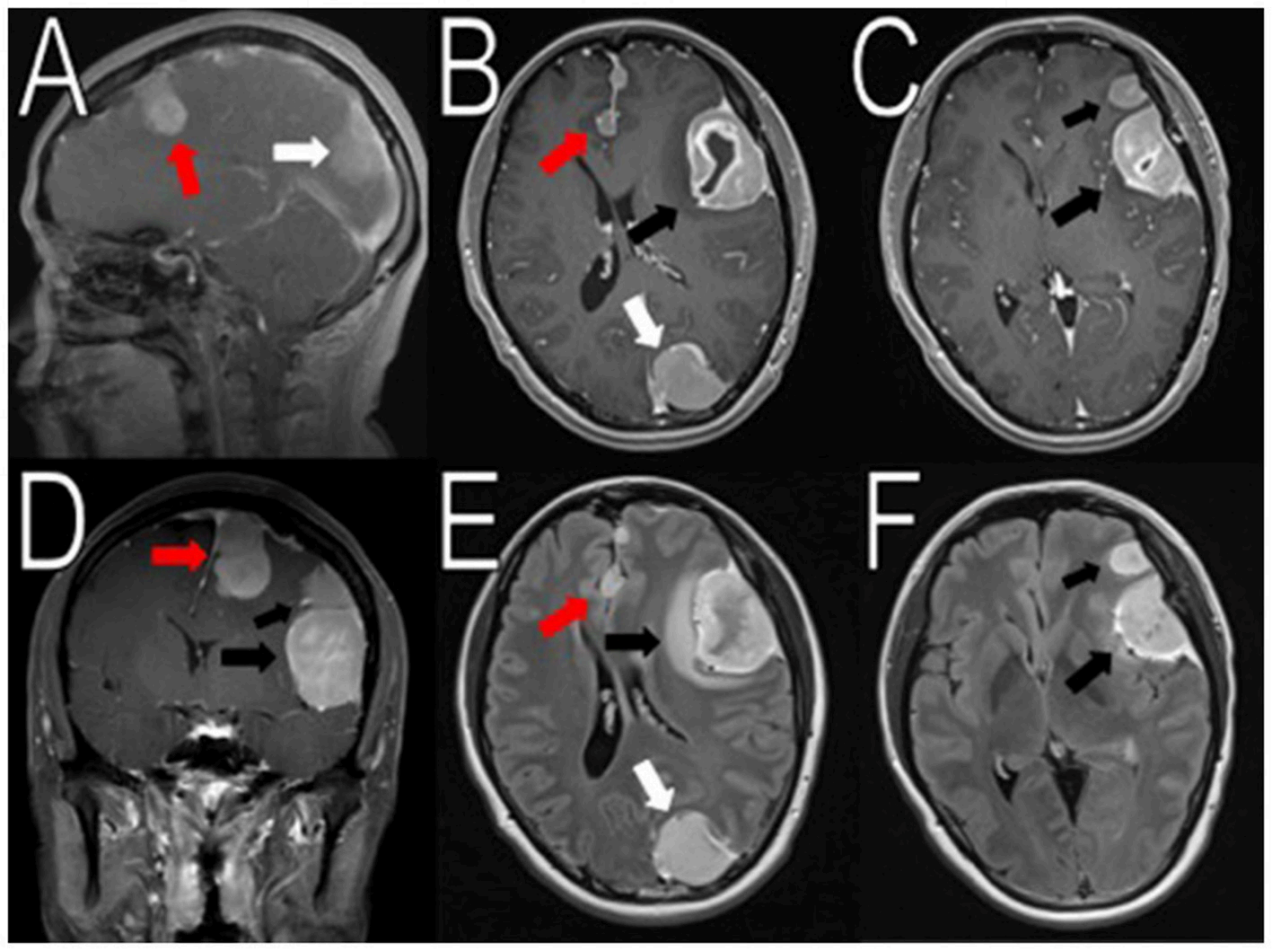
Initial imaging showing multiple menigniomas. Preoperative contrasted T1 and T2 MRI scans reveal multiple meningiomas. The left calcified parafalcine lobulated mass (2 × 2.2 × 3.3 cm, AP, TV, CC) was associated with vasogenic edema (red arrows in A,B, D–E). In the left frontal temporal convexity, there was a 4.5 × 2.9 × 4.1 cm (AP, TV, CC) mass with another 2.3 × 2.0 × 1.9 cm (AP, TV, CC) mass located superior to it (black arrows in B–F). In the occipital lobe, the mass was measured to be 2.6 × 2.9 × 3.9 cm (AP, TV, CC) (white arrows in A,B,E). There was an 8 mm rightward midline shift. The images seemed to suggest neurofibromatosis type 2.
